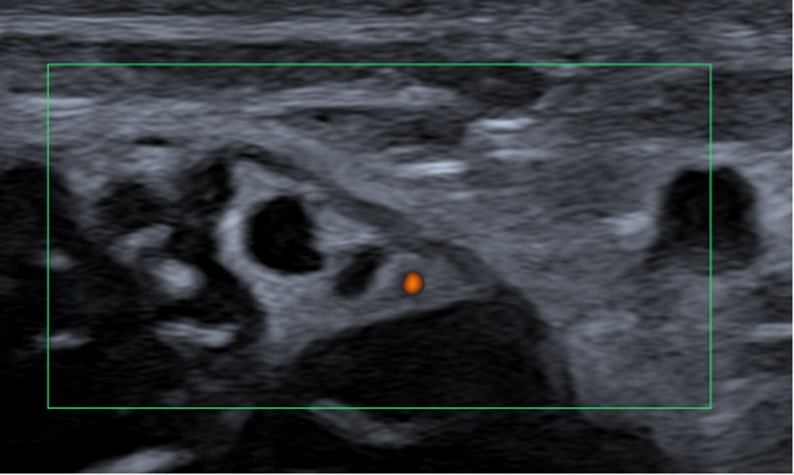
Axial section of power Doppler ultrasound, highlighting arterial vascularization adjacent to the ulnar nerve
